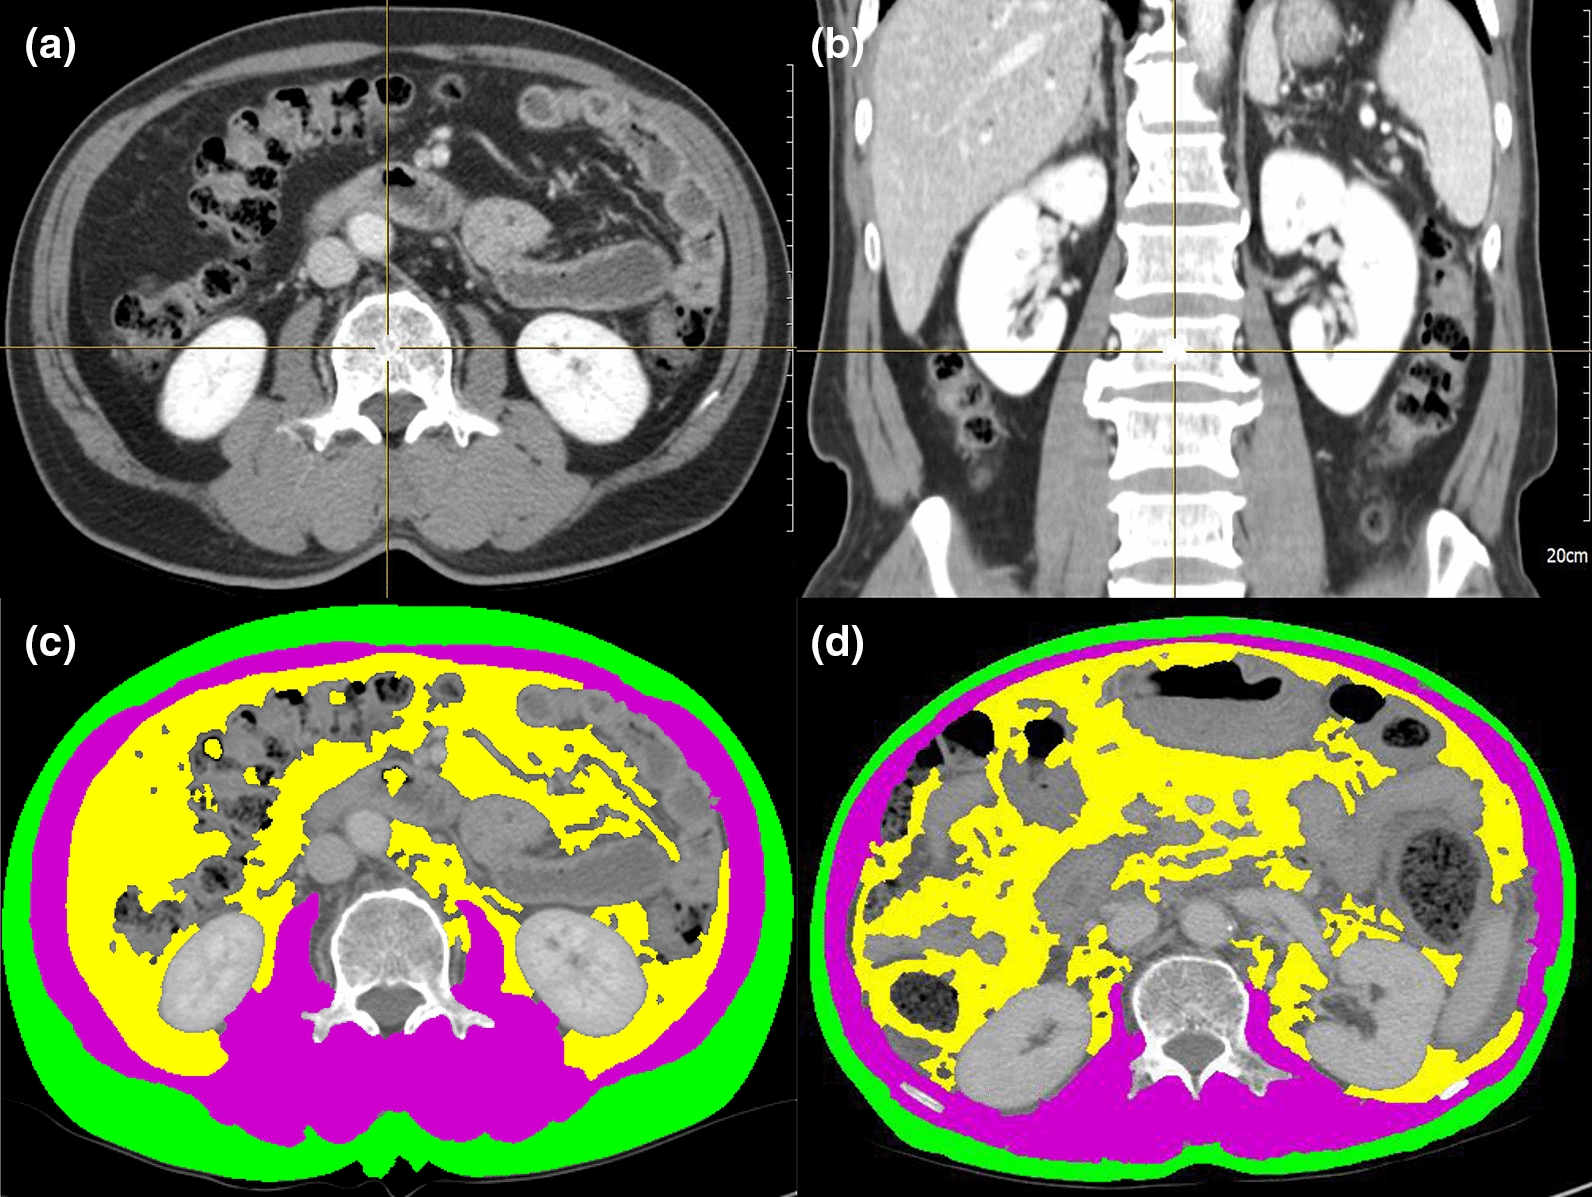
The quantification of body composition using computed tomography (CT) scan at the level of third lumbar vertebra (L3). a, b Cross-sectional image at L3 level that measured the skeletal muscle mass and fat mass. c CT image before FOLFIRINOX chemotherapy. d CT image after 6 months of FOLFIRINOX chemotherapy. The purple color indicates skeletal muscle, yellow indicates visceral adipose tissue, and green indicates subcutaneous adipose tissue
